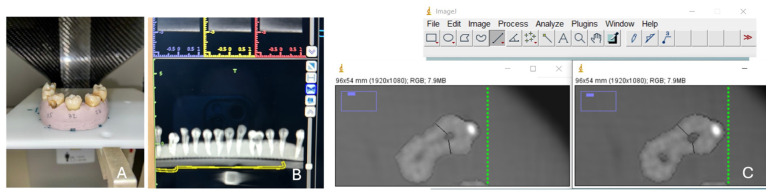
(A) Positioning of teeth for CBCT; (B) all teeth in the CBCT image; (C) ImageJ software distance measurements before and after instrumentation, according to the method of Gambill et al. [21] (black lines represent the distance from the wall of the canal to the external wall of the root in the mesial and distal directions).
